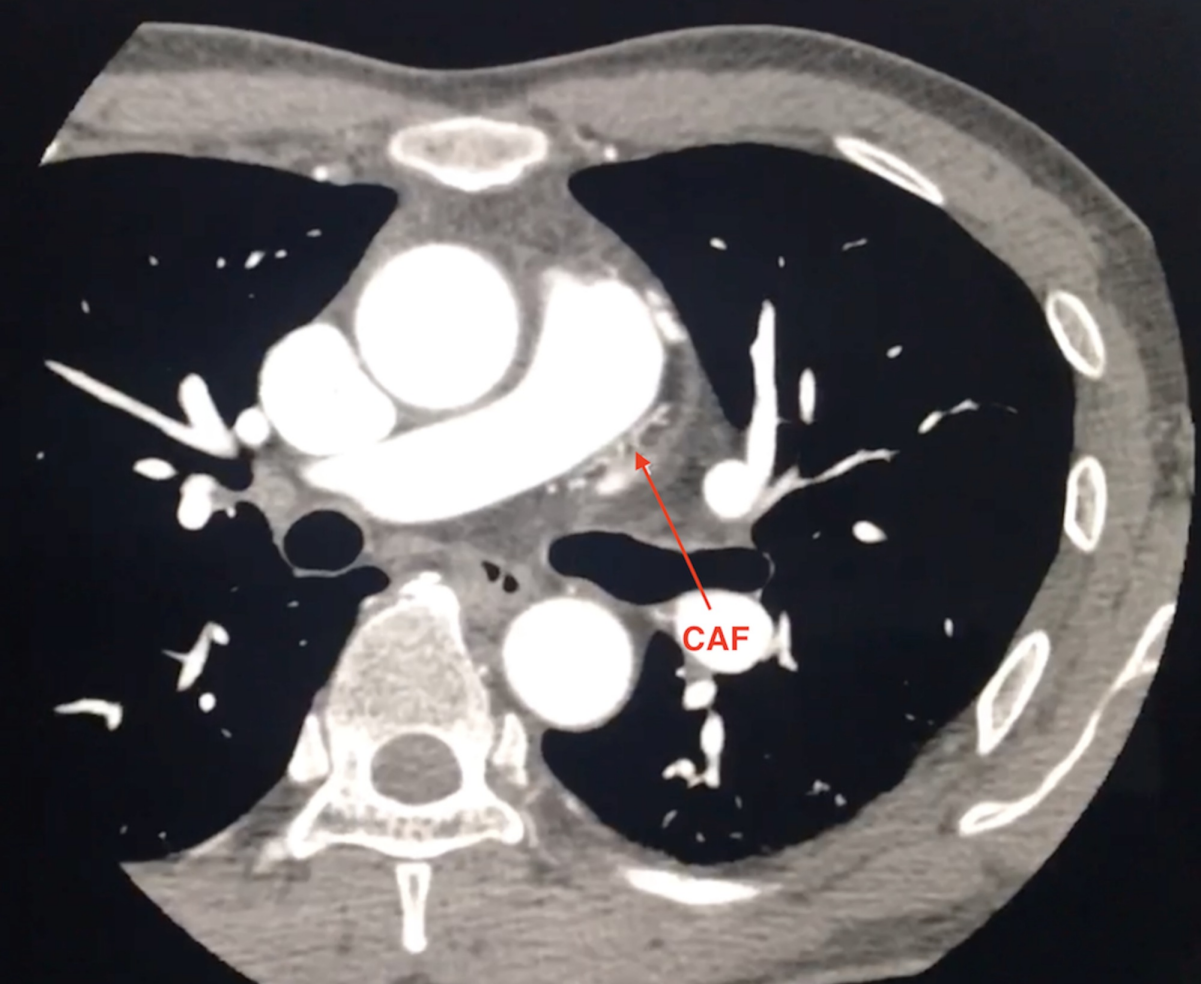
Axial CTA: Network of vessels seen along the PA trunk with loss of intervening fat plane suggestive of a fistulous connection CTA, computed tomography angiography; PA, pulmonary artery
